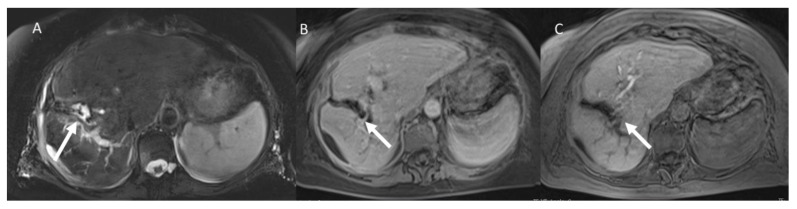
The same patient as Figure 5. MRI assessment. (A) SPACE T2-weighted sequence, (B): portal phase of contrast study and (C) EOB-phase after 1-month, arrow shows biloma and no bile leak.
